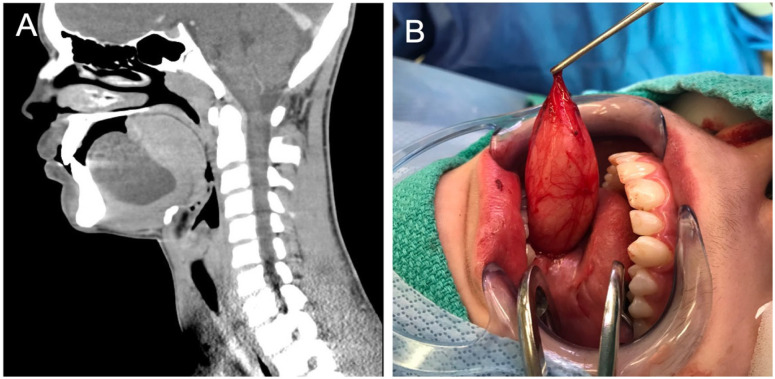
CT image and intraoperative image of the sublingual mass. (A) Sagittal CT view showing a ~5 cm × 5 cm sublingual mass. (B) Case 2: Intra-operative image of sublingual mass excision. Dermoid cyst measured ~5 cm × 5 cm.
